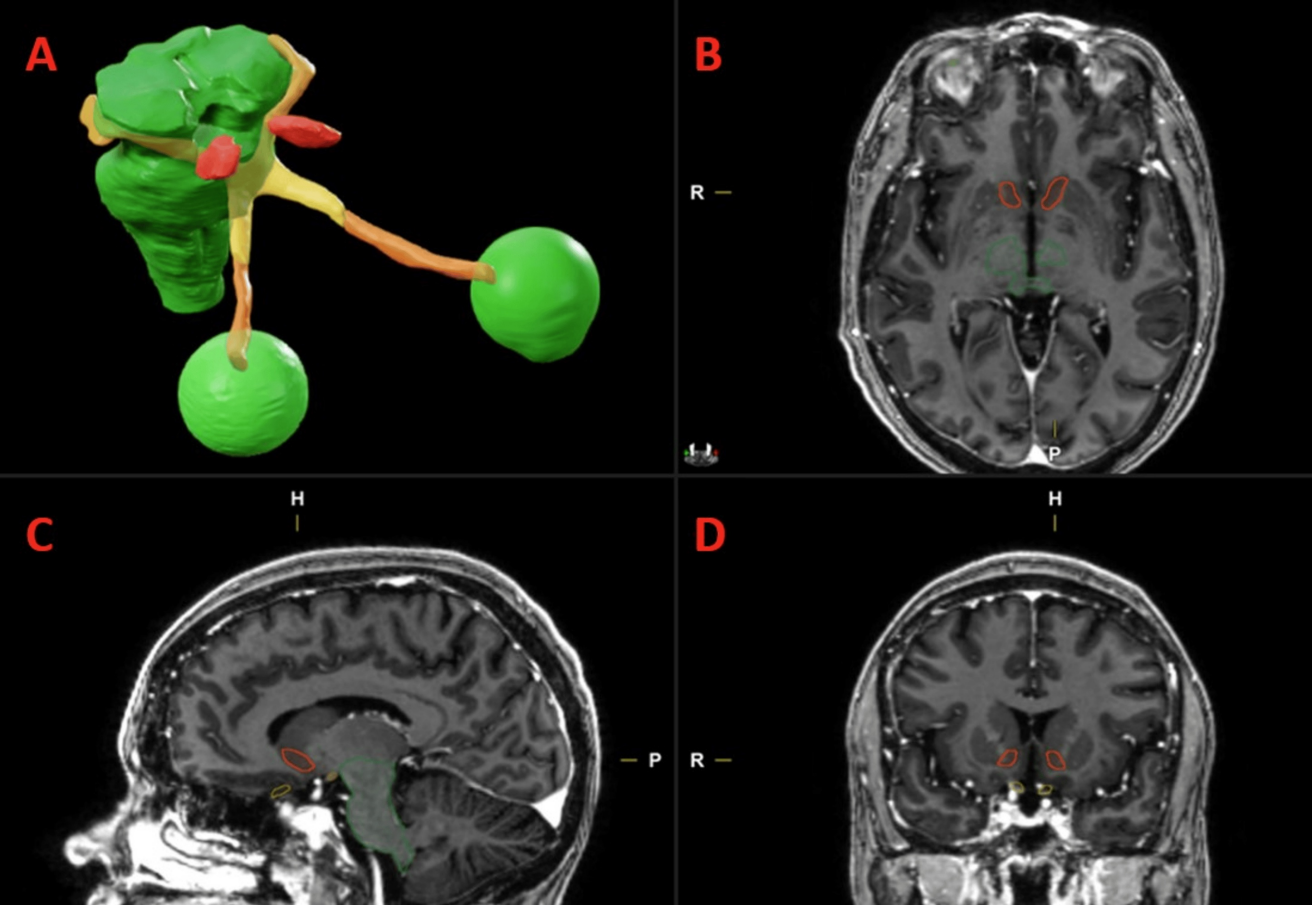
Critical structures Volumetric representations of brainstem (green); optic nerves, chiasm, tract (yellow/orange); eyes (green); and nucleus accumbens (red) (A). Volumes displayed on test patient MRI in axial (B), sagittal (C), and coronal (D) views.
